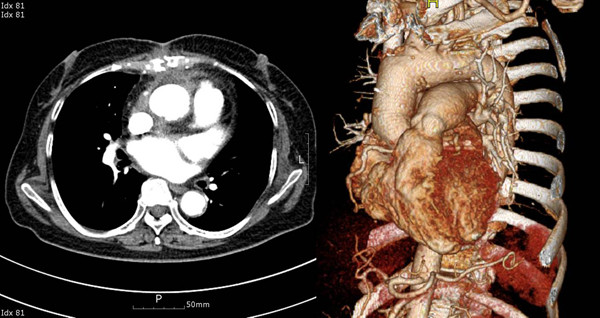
The Postoperative chest CT scan shows the ascending aortic graft without pseudoaneurysm.
